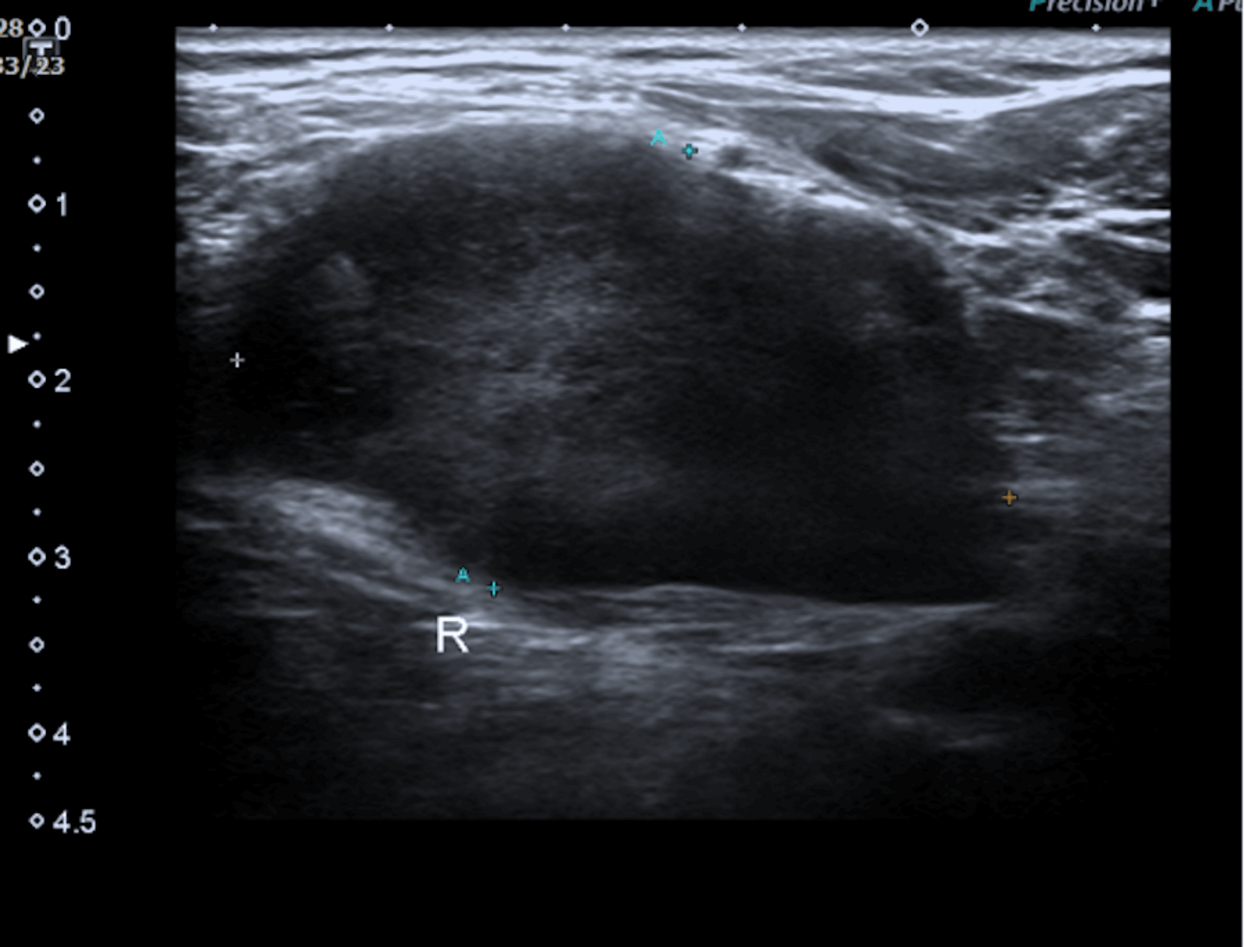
An ultrasound of the neck revealed a large, well-defined, heterogeneous, hypoechoic lesion measuring 2.7 cm × 4.4 cm (AP × W) seen within the right sternocleidomastoid muscle. AP × W: anteroposterior length × width
